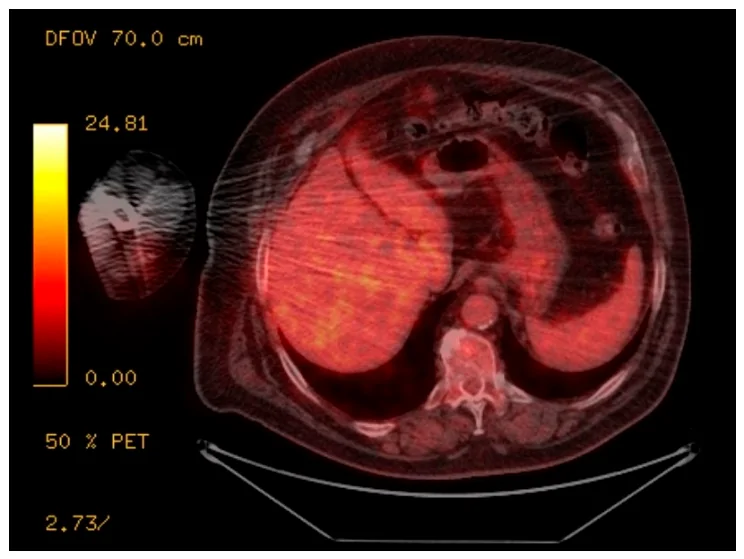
Preoperative axial fused PET/CT image at the level of the upper abdomen.
